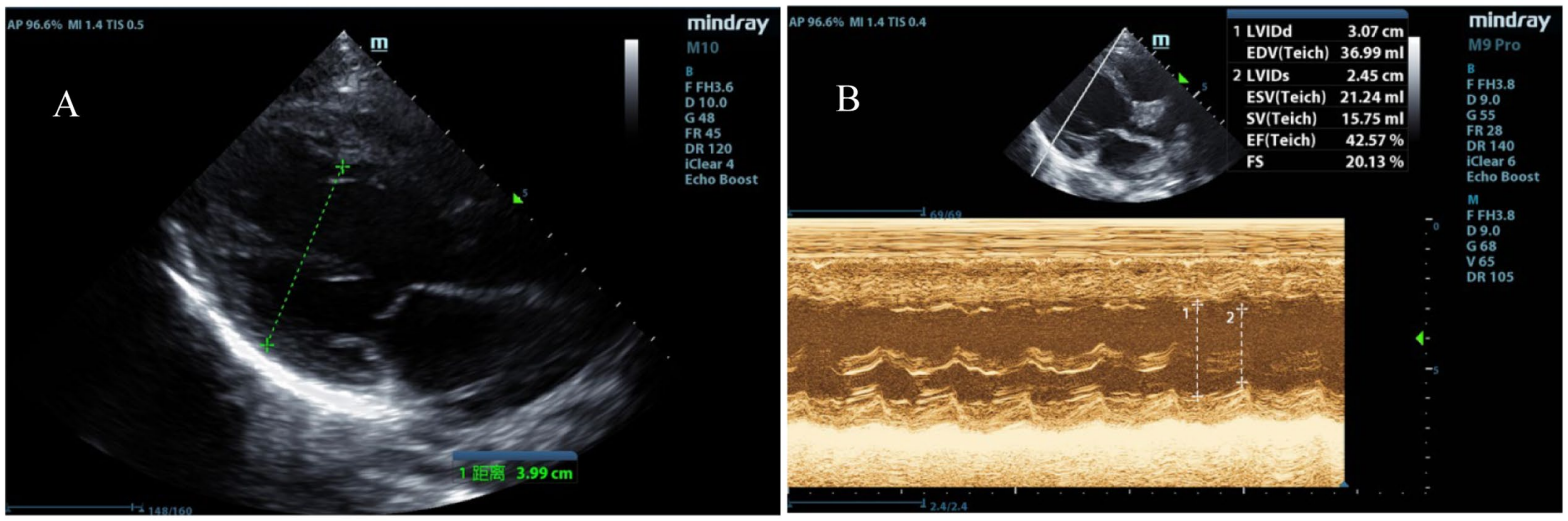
Echocardiographic indicators of left heart failure. (A) Enlargement of the left ventricular chamber. (B) Reduced left ventricular systolic function with an EF of 42% and FS of 20%.
